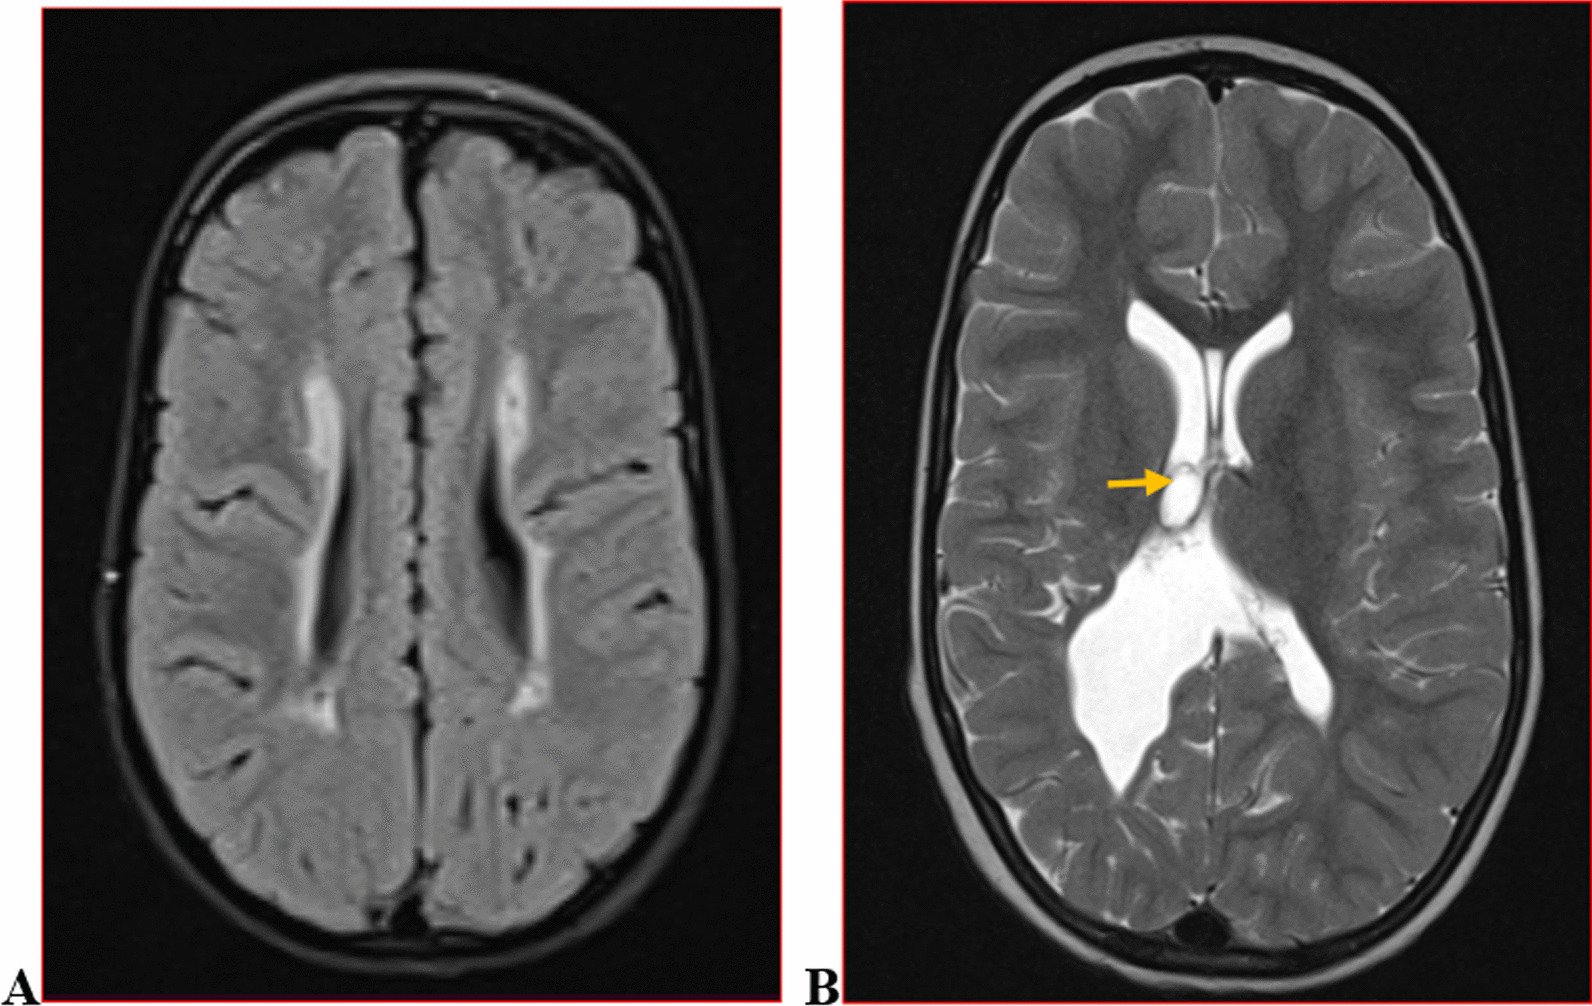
Typical PVL (A) with symmetrical FLAIR hyperintense periventricular rim representing periventricular gliosis with associated thinning of the white matter. Atypical PVL (B) assymetrical thinning of white matter with apparent dilation and irregular outline of the trigone and the posterior horn of the right lateral ventricle. Note is made of a right intraventricular cyst(arrow) which was an incidental finding
